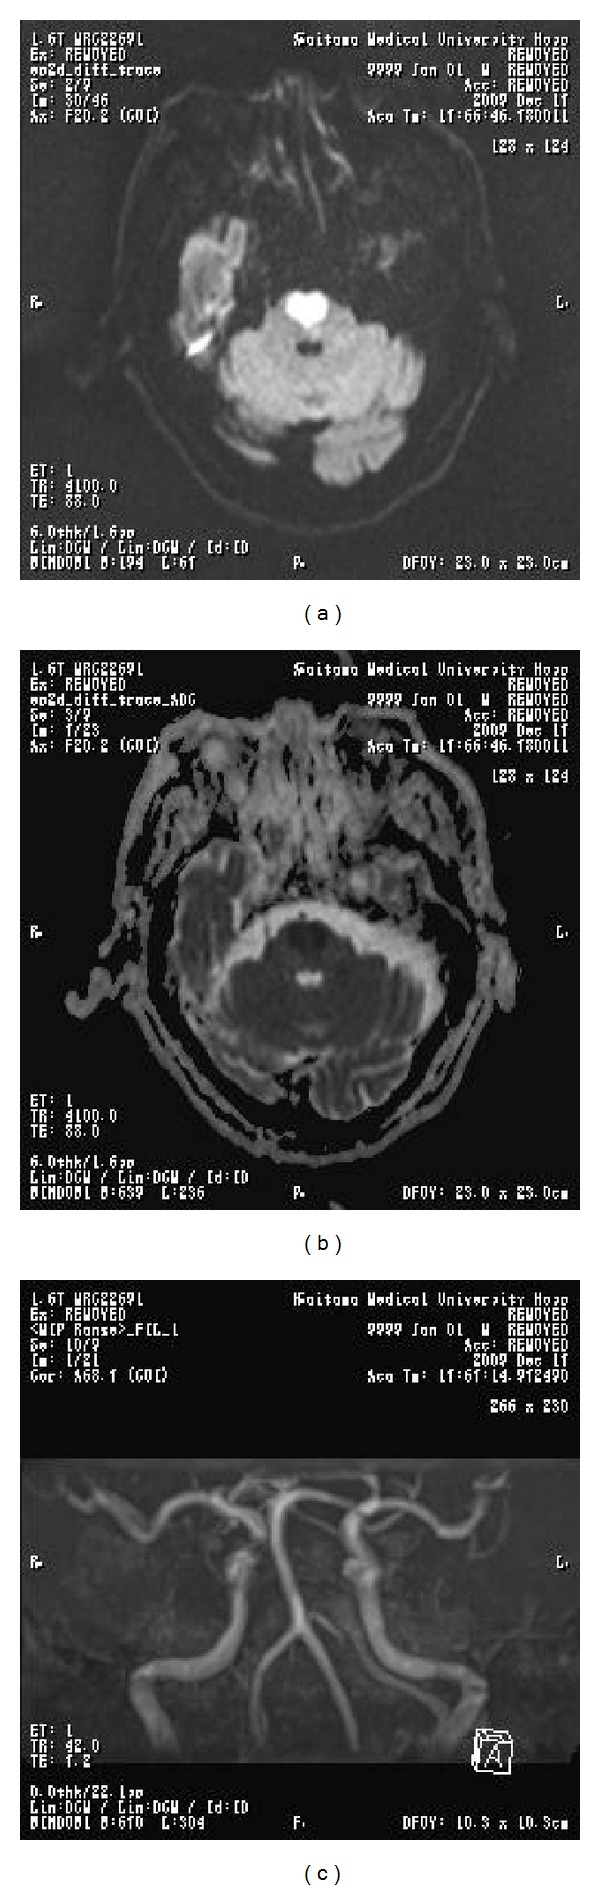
(a) The brain diffusion-weighted image MRI (DWI) of the patient is shown. Note the “heart appearance” lesion showing high intensity in the pontine base. (b) The brain apparent diffusion coefficient map MRI (ADC) of the patient is shown. The “heart appearance” lesion shows low intensity on ADC, indicating, in conjunction with the finding on DWI, that the lesion is a fresh infarct. (c) The brain magnetic resonance angiography (MRA) of the patient is shown. The basilar artery remains intact despite the bilateral involvement of the pons.
